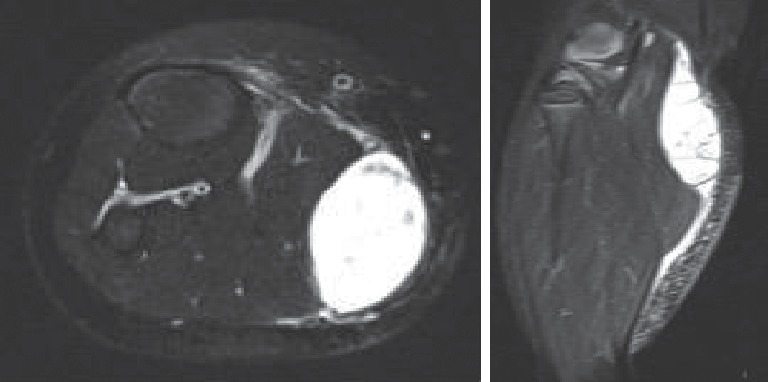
MRI of Baker's cyst of the case with partial rupture in the superfical posterior compartment.
